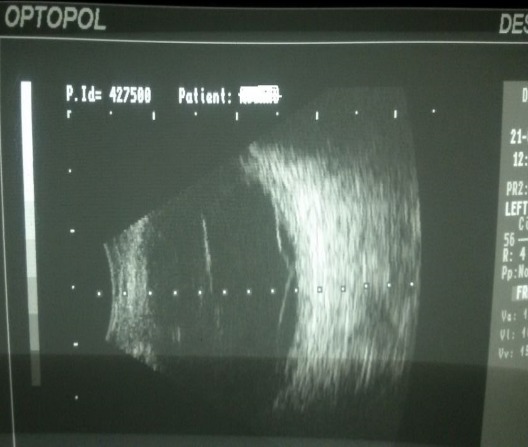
B-scan ultrasonography OS
